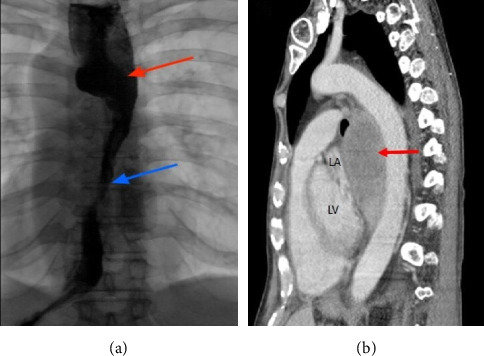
(a) Barium swallowing radiogram showing proximal esophageal dilation (red arrow) and midesophageal narrowing (blue arrow). (b) Chest computed tomography, sagittal section through the left atrium showing significant left atrial compression from an esophageal mass (red arrow) (adapted from Polina et al.) [12]. Abbreviations: LA: left atrium; LV: left ventricle.
